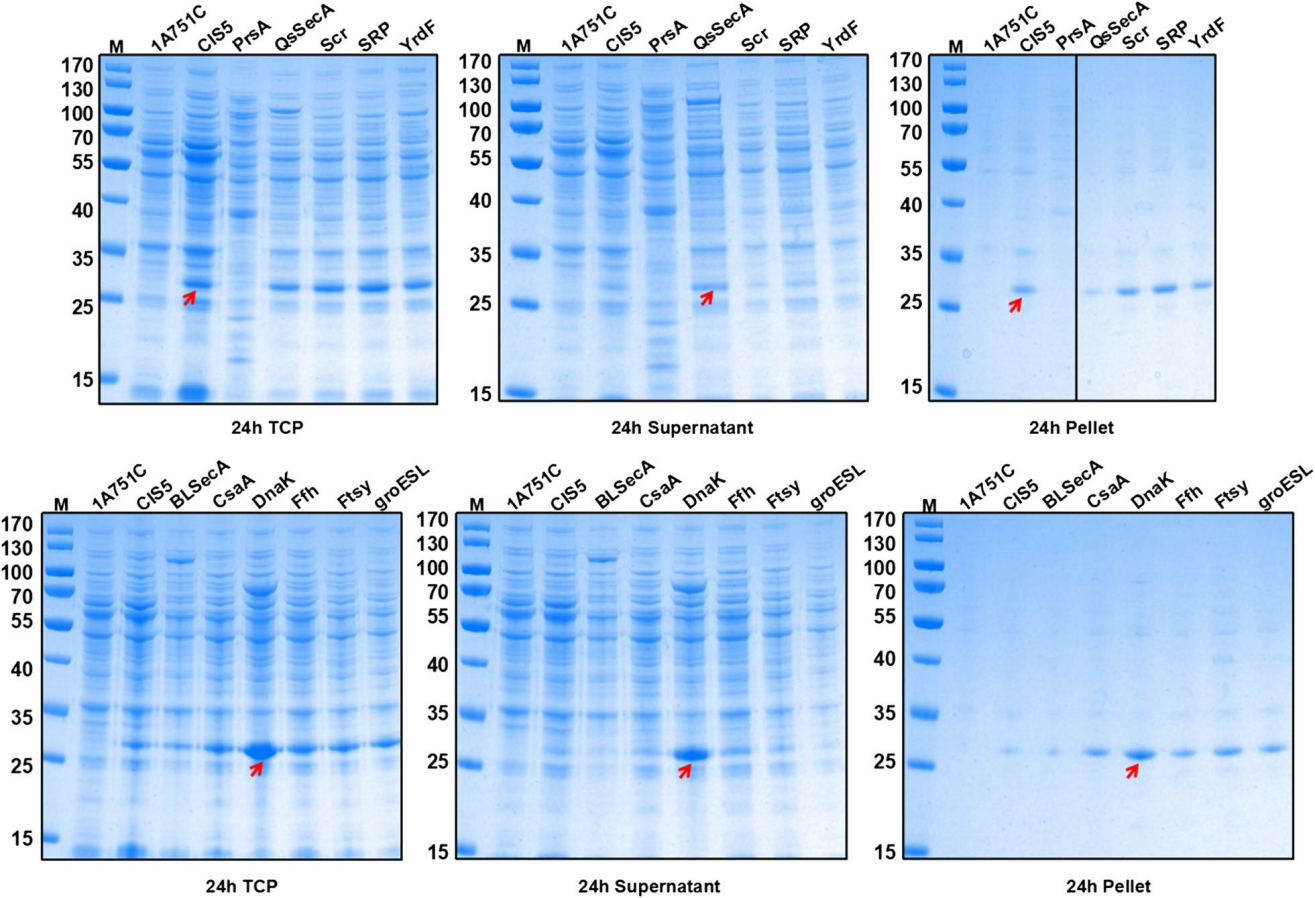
SDS-PAGE analysis of rhFGF21 expression following ultrasonication. Total cell protein (TCP), supernatant, and pellet fractions from *B. subtilis* strains that overexpressed different chaperones at incubation of 24 h were run. Strains *B. subtilis* CHAP1, CHAP2, CHAP3, CHAP4, CHAP5, CHAP6, CHAP7, CHAP8, CHAP9, CHAP10, and CHAP11 overexpressed *blsecA*, *csaA*, *dnak*, *ffh*, *ftsY*, *groESL* operon, *prsA*, *qssecA*, *scr*, *SRP,* and *yrdF*, respectively. The molecular weight of mature rhFGF21 is about 27 kDa. Column M: molecular weight marker. The strains 1A751C and CIS5 served as negative and positive controls
